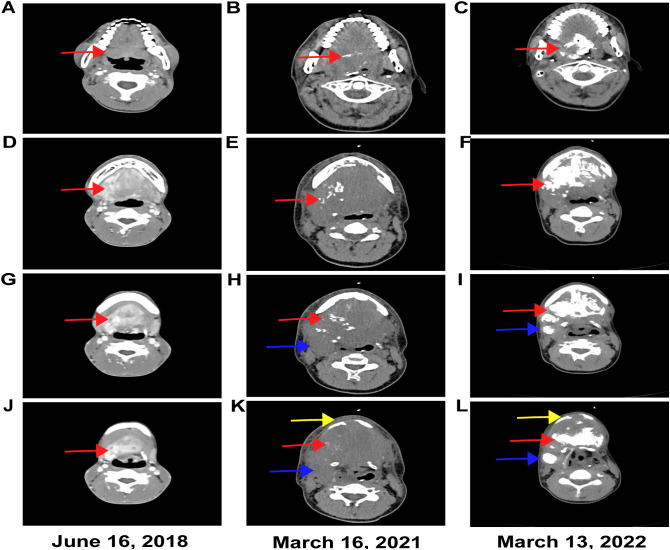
As demonstrated in the CT images labeled (A, D, G, J), which were captured on June 16, 2018. A clump-like soft tissue density shadow was identified in the right mandibular angle region. Heterogeneous enhancement was observed on the enhanced scan images. This marks the first recurrence of the tumor. There was an absence of calcification in both the tumor region and the lymph node drainage area. As showed in the CT images labeled (B, E, H, K), which were conducted on March 16, 2021. In the right mandibular angle region, an irregular soft tissue density mass measuring approximately 88 × 79 × 65 mm was identified. This mass demonstrated multiple punctate calcifications and was associated with destructive changes in the adjacent bone. This moment occurred after the completion of palliative radiotherapy and prior to the initiation of treatment with anlotinib in combination with toripalimab for the second relapse. As showed in the CT images labeled (C, F, I, L), which were conducted on March 13, 2022. A mass measuring up to 84 mm in maximum diameter was identified in the right mandibular angle, associated with significant calcification and adjacent bone destruction. The red arrow indicated tumor extension from the base of the tongue to the floor of the mouth. The yellow arrow highlighted mandibular destruction, and the blue arrow denoted lymph nodes located in the Ib region.
